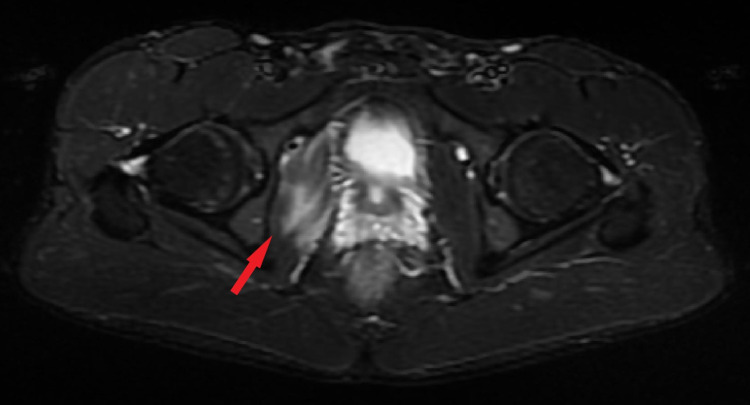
MRI STIR Sequence Axial View Axial view of the right hip at the level of the greater trochanter.  High signal (Grade II muscle strain) in the obturator internus muscle belly at the intrapelvic route. No other pathology was revealed. The red arrow shows the obturator internus. STIR: Short Tau Inversion Recovery Image.
